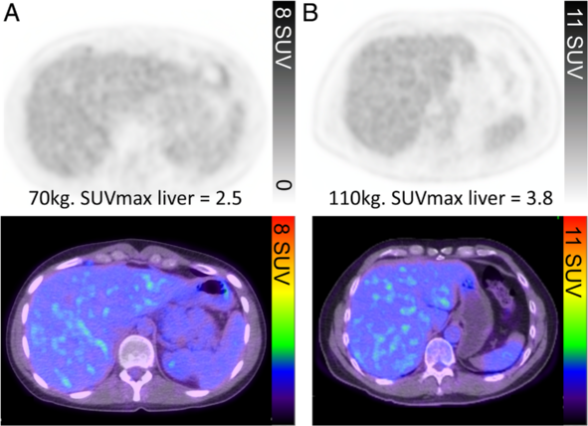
The PET window intensity is adjusted so that the liver appears light to mid-grey on the grey scale, corresponding to flecks of green in the liver on the rainbow colour scale. Despite the difference in SUVmax of the liver secondary to differences in weights of the two patients (a and b), the liver intensity this appears the same in both patients
